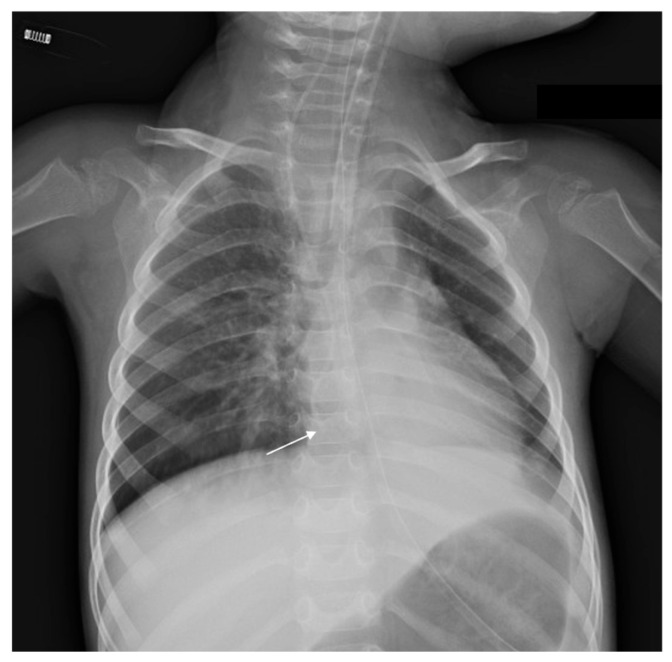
Chest radiography of Patient 2—on day 3 of hospitalization, S-shaped folding of the catheter tip can be visualized, raising the suspicion of dislocation (white arrow).
